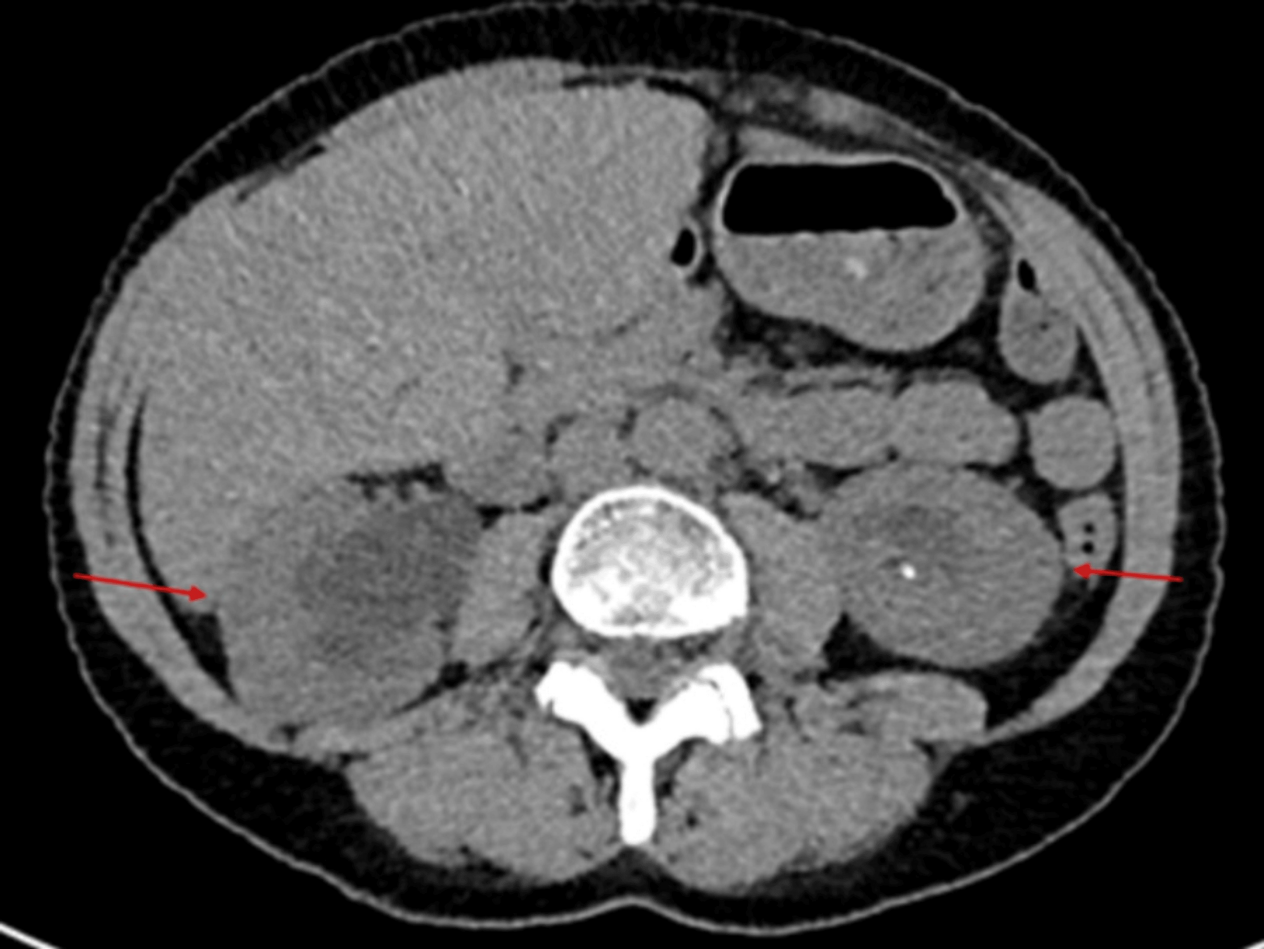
CT of the kidneys, ureters, and bladder showing bilateral moderate hydronephrosis (red arrows) secondary to retroperitoneal fibrosis.
